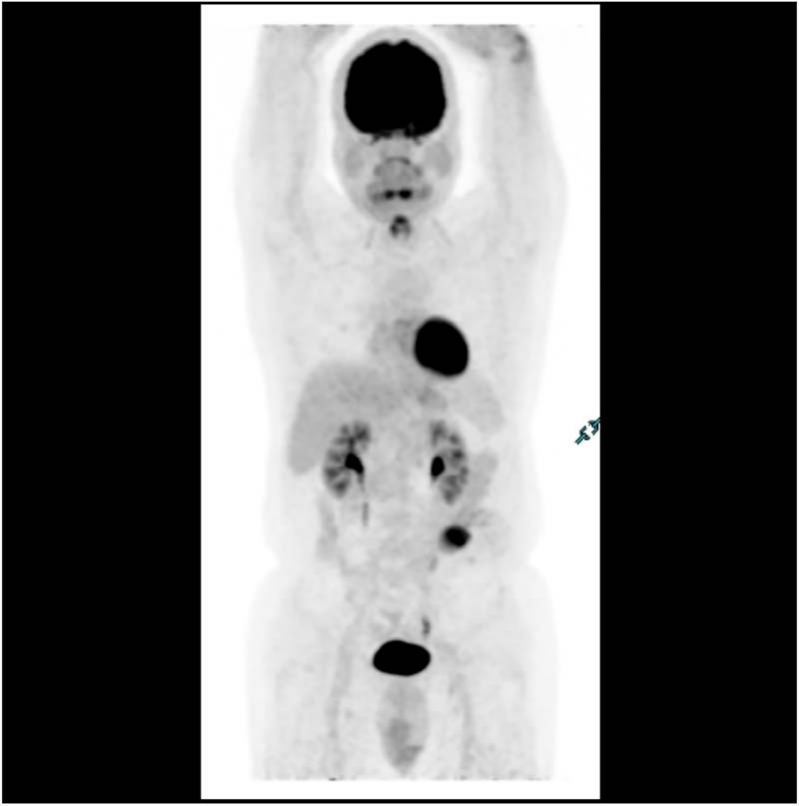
PET scan in 2018 demonstrating no evidence of FDG avid regional nodal or distant metastasis, especially in the liver.
